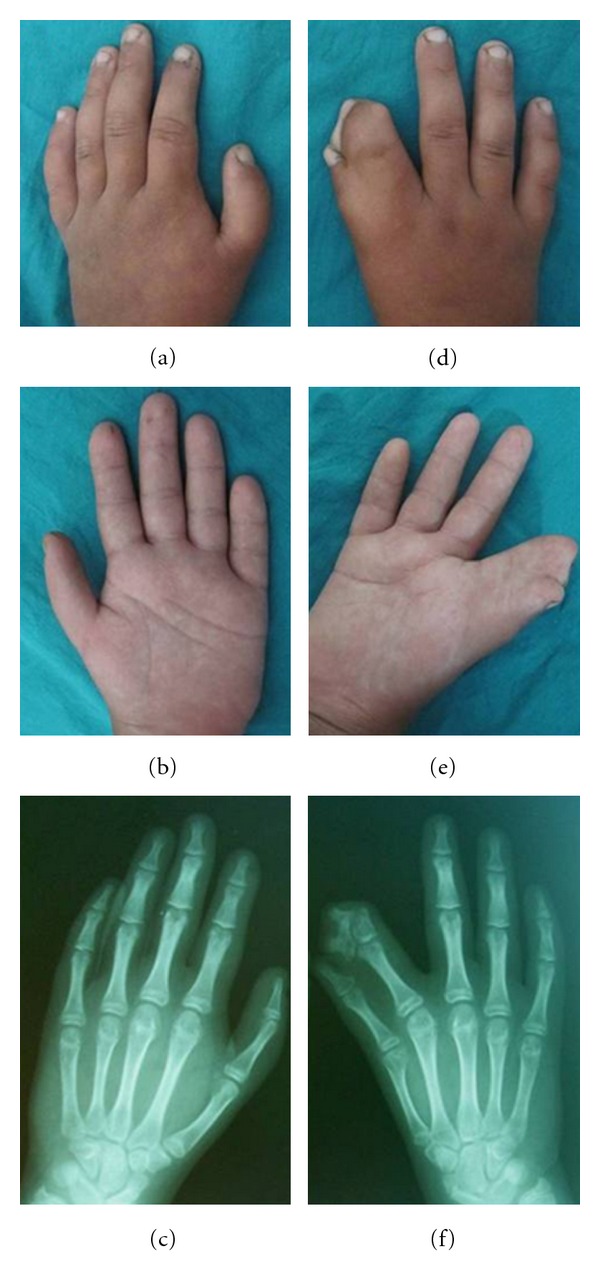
Limb abnormalities in the patient's uncle. Hands photographs and radiographs showing (a)–(c) hypoplastic thumb in the left hand and (d)–(f) syndactyly between the first and second fingers, with an extra nail in the right hand.
